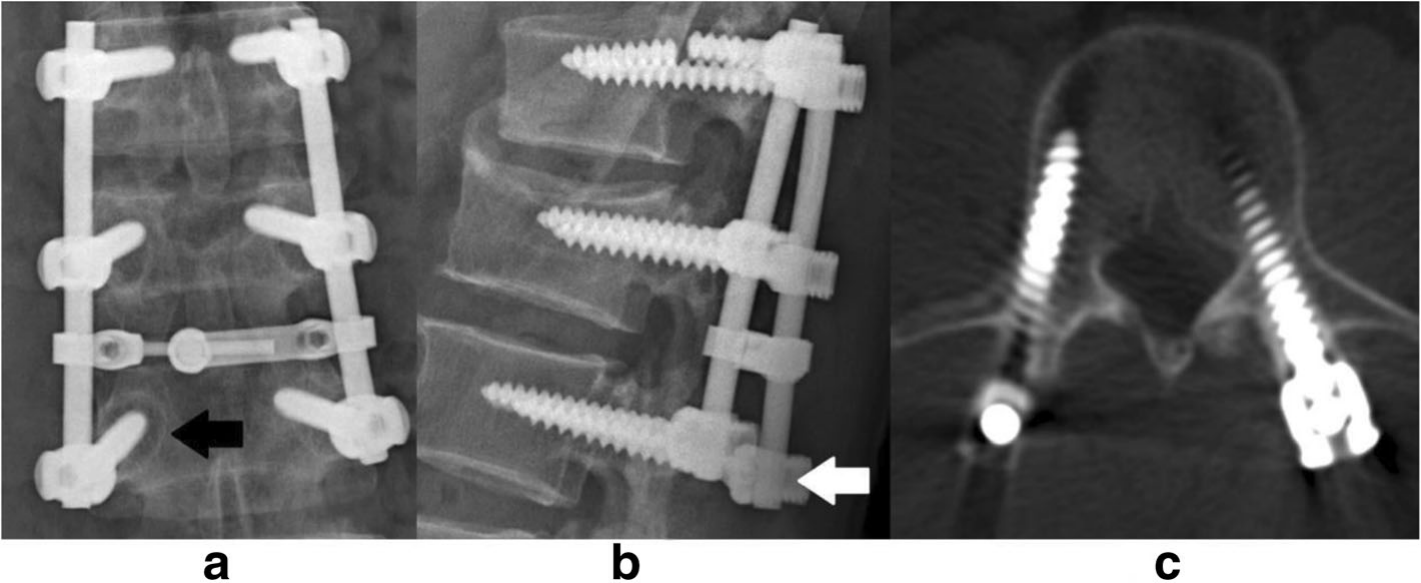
A typical case showing CT scan might be insensitive to detect screw loosening. a Anteroposterior X-ray showed radiolucent zone and double halo around the screw (black arrow) which indicate screw loosening. b Lateral X-ray showed that the same screw was pulled out (white arrow). c CT scan showed no gap around the same screw
